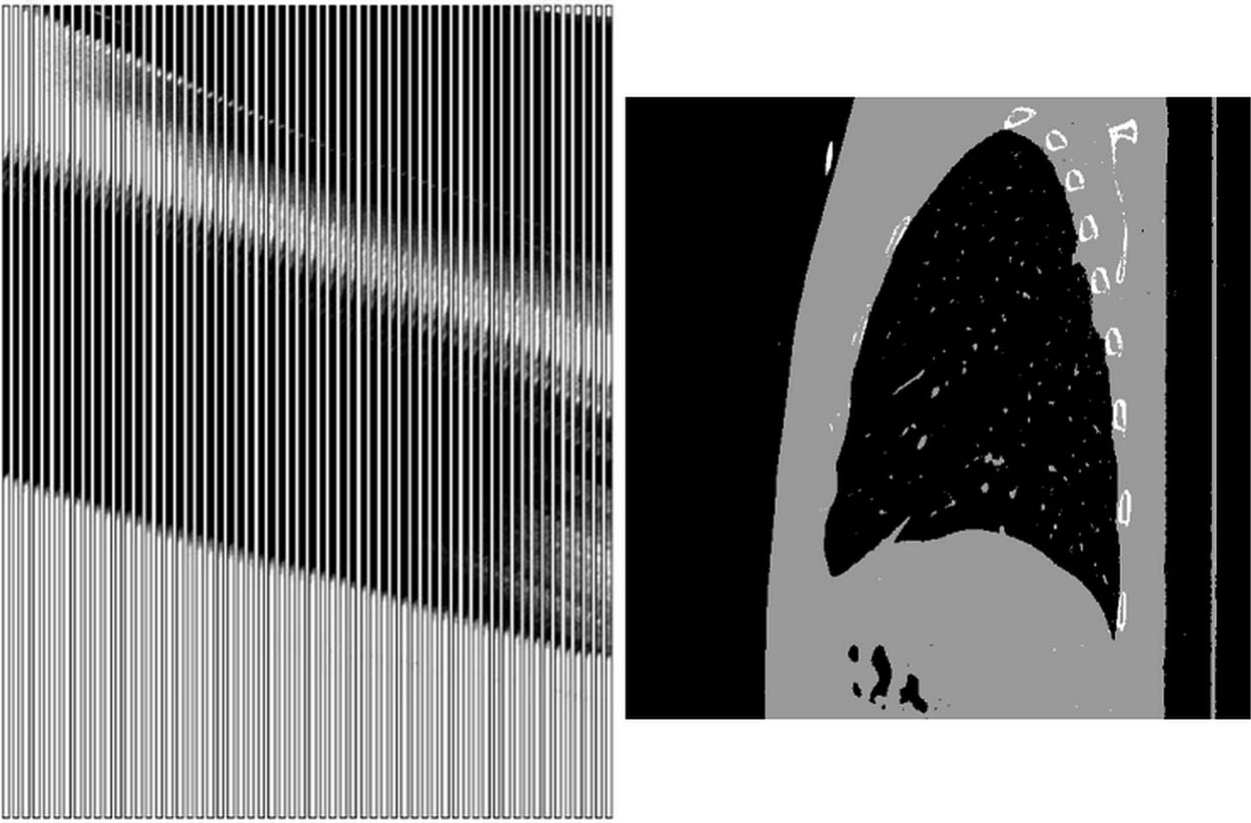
An example input (60 projection patches, left) output (corresponding sagittal CT slice, right) pair. Due to the generation approach, they can now be used for supervised training.
